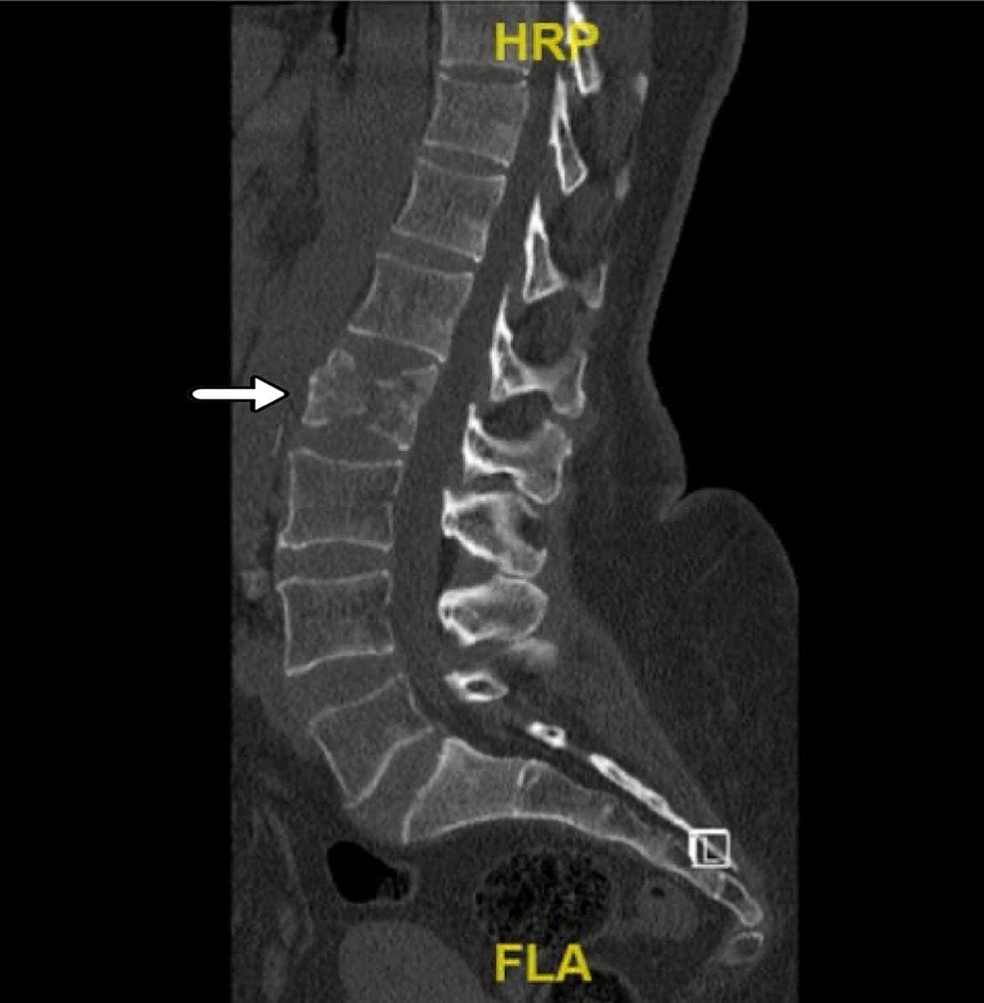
CT scan lumbar without contrast Showing L2 vertebral body compression fracture with no definitive compression on the spinal cord
